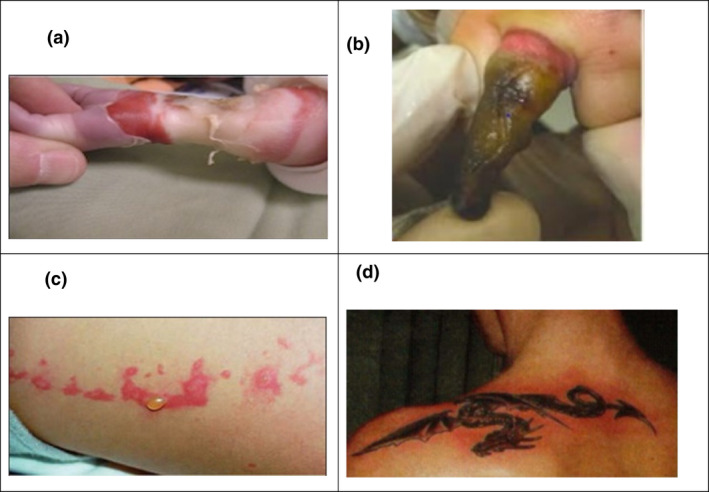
Examples of RF induced burns: (A) fourth‐degree burn immediately after MRI examination on a 5‐week‐old baby, caused by an MR Unsafe pulse monitor. This resulted in amputation of the forearm^58^ (B) burn resulting from an MR Unsafe pulse oximeter on the finger, post‐escharotomy (C) second‐degree burn after MRI examination from invisible metallic microfibres in clothing (D) first‐degree burn around a tattoo after MRI. (Images courtesy of, and reprinted with permission from, Frank G. Shellock, Ph.D., www.MRIsafety.com).
